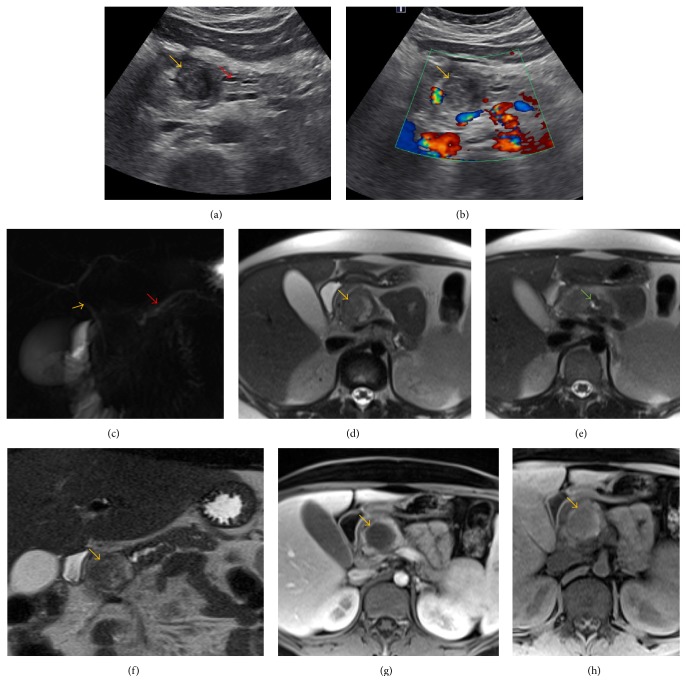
(a and b) Ultrasound images. (a) Well-defined hypoechoeic lesion without necrosis or calcification (orange arrow). Mildly dilated pancreatic duct (red arrow). (b) Color Doppler evaluation showing no color flow within the lesion in the pancreas (orange arrow). (c, d, e, f, g, and h) MRI and MRCP images. (c) MRCP-Normal CBD (orange arrow). Mildly dilated pancreatic duct (red arrow). (d) T2 weighted axial image showing heterogeneous mass in the head of the pancreas (orange arrow). (e) Mildly dilated pancreatic duct (green arrow). (f) Coronal T2 weighted image showing heterogeneous mass in the head of the pancreas (orange arrow). (g) Axial T1 weighted image without contrast showing well-defined hypointense lesion in the pancreas (orange arrow). (h) Axial postcontrast image showing no enhancement of the lesion in the head of the pancreas (orange arrow).
